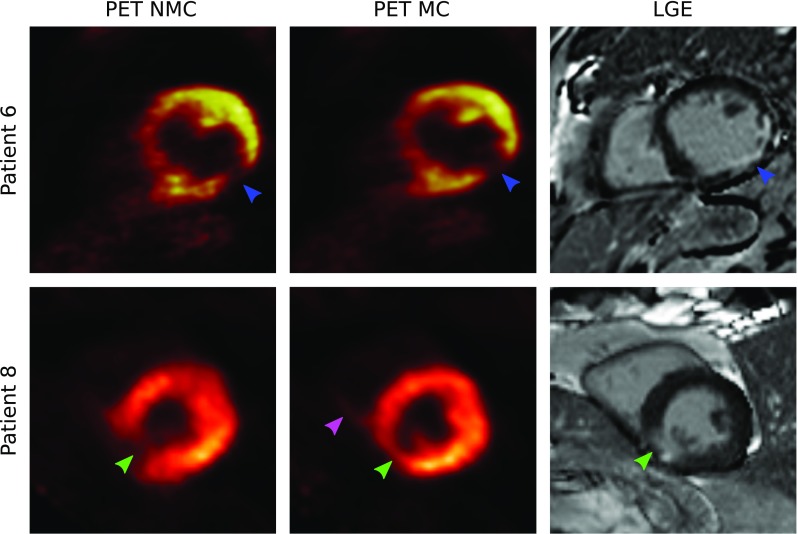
Short axis view for two representative patients (*rows*) showing uncorrected (NMC) and motion-corrected (MC) viability ^18^F-FDG PET images, and corresponding 2D LGE scans. MC improves the correspondence of the PET images to the anatomy as observed in the LGE images, particularly in the delineation of viability defects (*green and blue arrows*) and right ventricle myocardium (*pink arrow*)
